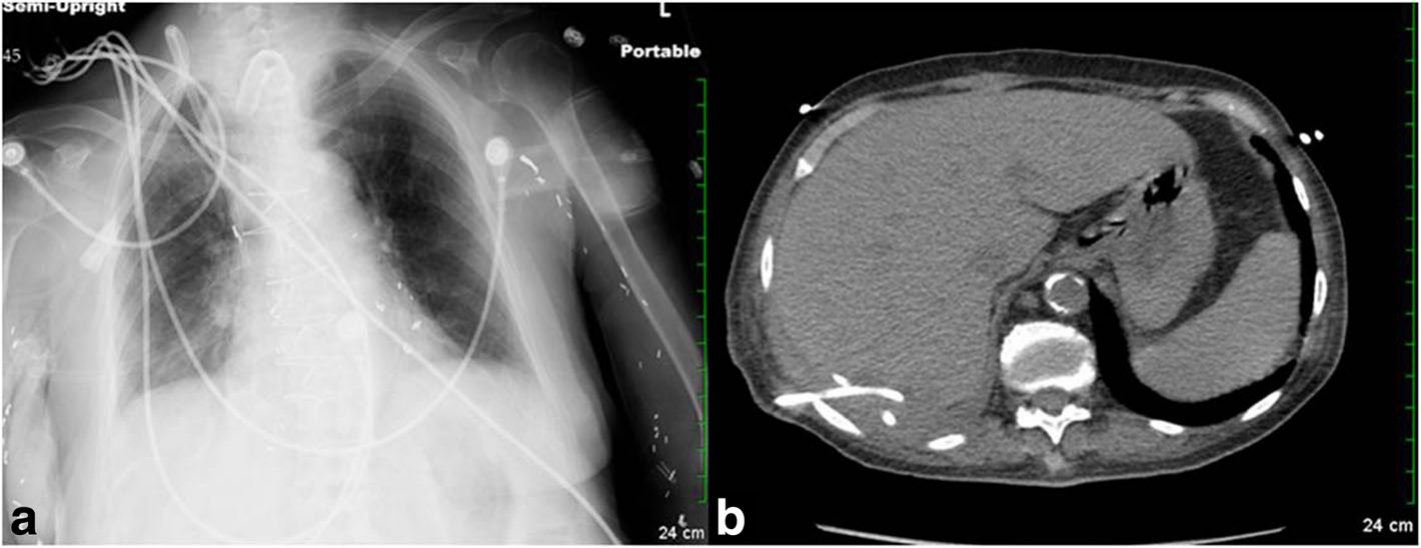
a CXR after treatment shows significant improvement comparing to CXR in Fig. 1a. b CT scan of abdomen shows significant improvement with pigtail catheter in place
